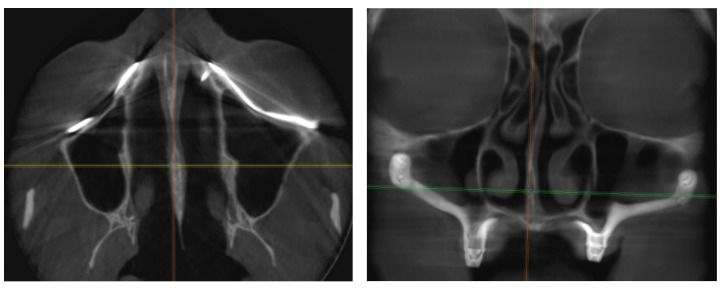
Radiographic images of a properly fitted dental implant at the two-year follow-up.
